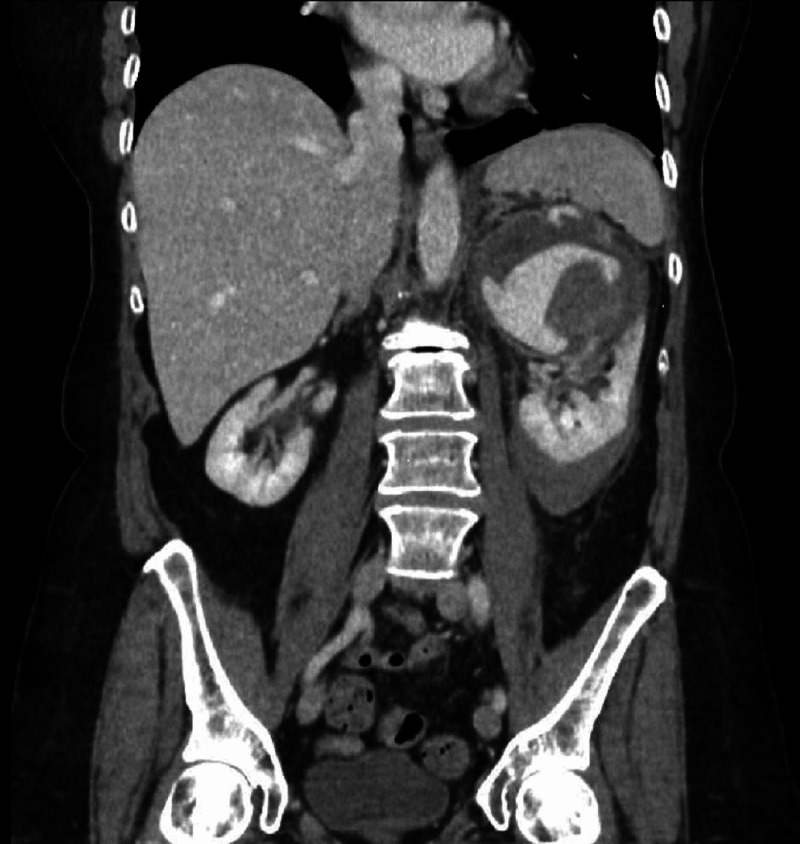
Coronal reconstruction of portal venous phase contrast-enhanced CT abdomen and pelvis demonstrates acute hemorrhage at the superior pole of the left kidney with active extravasation.
